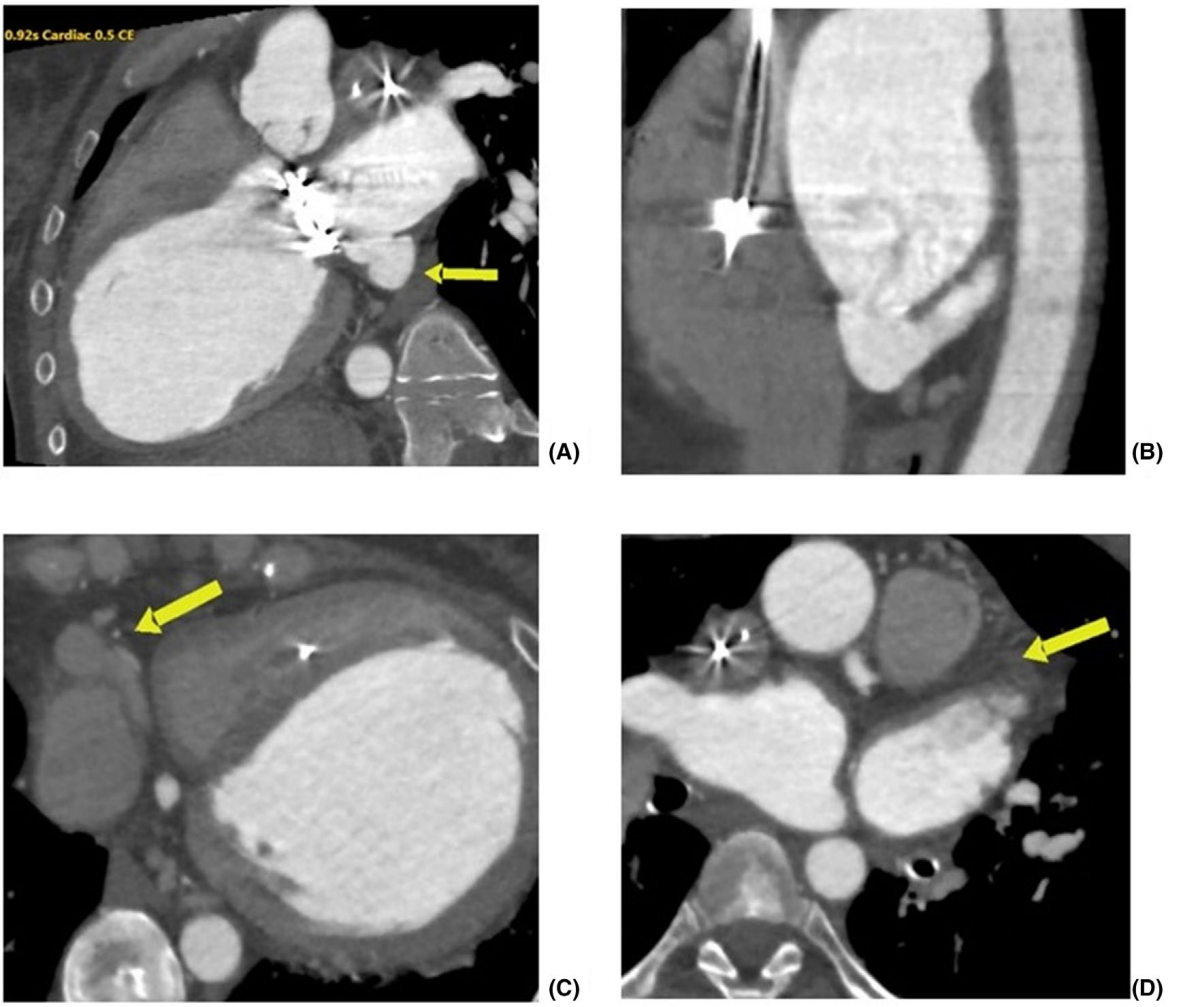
Computed tomography angiography (CTA), oblique multiplanar reconstructions. (A) Unroofed coronary sinus (yellow arrow), wide communication with left atrium. (B) Unroofed coronary sinus with abrupt discontinuation. No connection with right atrium is visible. (C) Enlarged cardiac veins draining into the right atrium (yellow arrow). (d) axial plane, no persistent left superior vena cava is present (yellow arrow)
